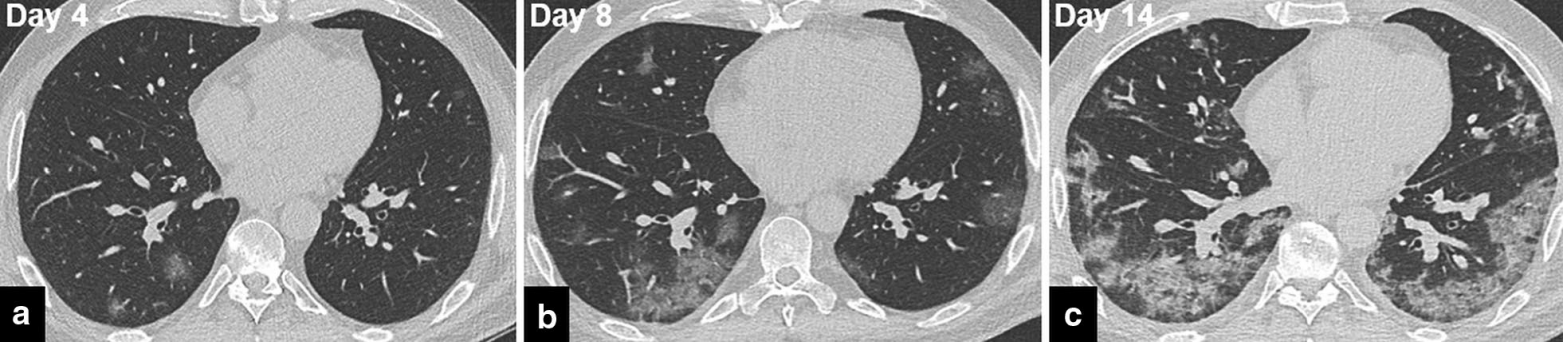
CT findings of COVID-19 pneumonia. a The first CT scan obtained on illness day 4 revealed patchy GGOs in both the lungs. b On day 8, the number and size of GGOs increased. c Consolidations were the dominant CT finding on day 14
